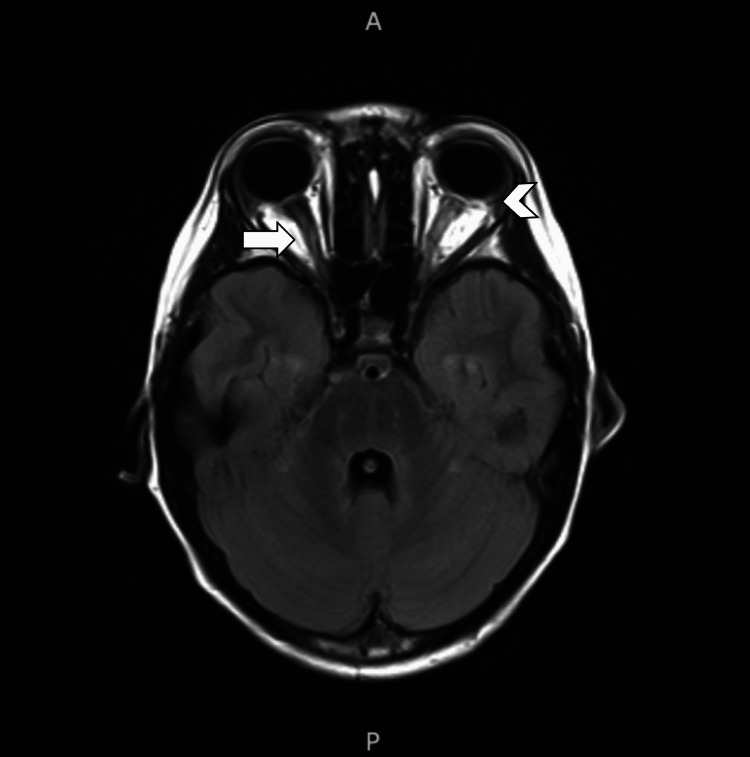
Brain MRI scan Demonstration of increased intracranial pressure on T1-weighted brain MRI scan, showing optic nerve sheath distension (arrow) and flattening of the posterior globes (arrowhead)
